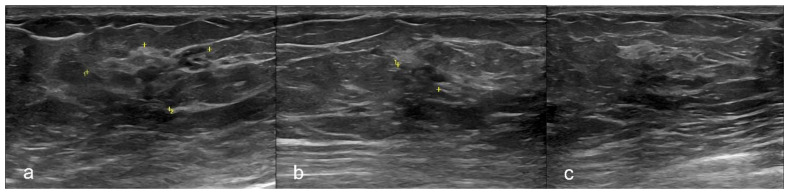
Ultrasonography. Breast ultrasound (US) in different projections showing an ill-defined, hypoechoic area of acoustic shadowing with peripheral anechoic lacunae at the union of outer quadrants of the left breast. (a) The yellow mark showed the whole area. (b) The yellow mark showed the small area of architectural distortion with acoustic shadowing. (c) US-guided Fine-Needle Aspiration Cytology (FNAC) of the lesion.
